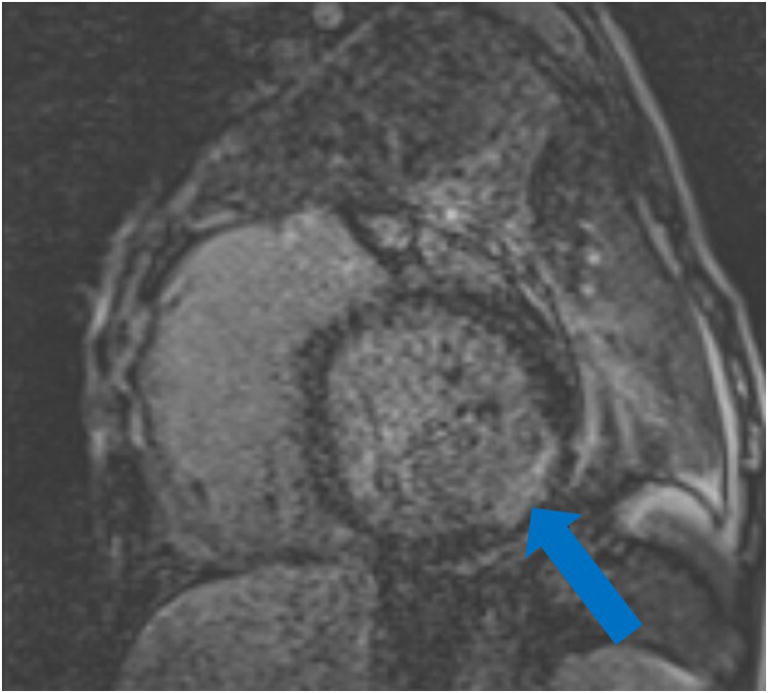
Myocardial infarction (MI) in a 56-year-old man: Two chamber post-contrast cardiac MRI shows transmural delayed enhancement consistent with MI involving the inferolateral base of the left ventricle.
